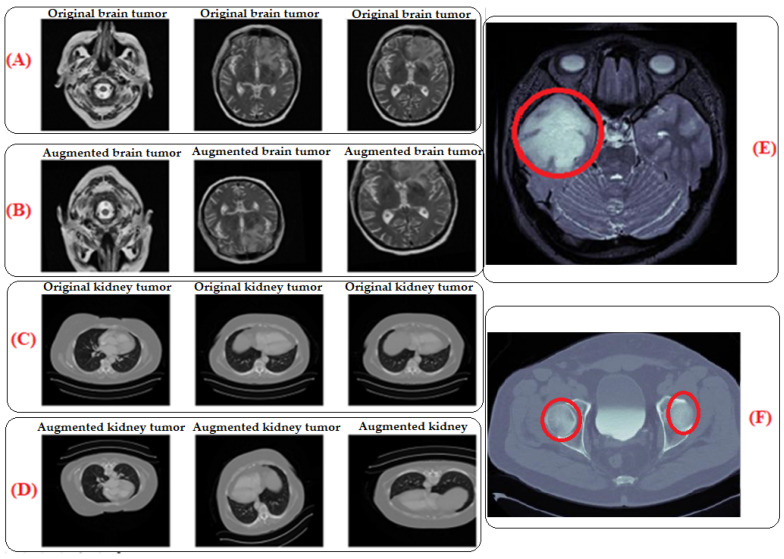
MRI images of original and augmented brain and kidney tumor data. The area circled in red color delineates the tumors. (A): Original brain tumor image (This represents the unaltered, initial image used in the training dataset, (B): Augmented brain tumor image (This corresponds to the image of the brain image that has undergone traditional data augmentation techniques), (C): Original kidney tumor image (This represents the original unaltered MRI scan focused on the kidney, (D): Augmented brain tumor image (This corresponds to the image of the kidney tumor, that has undergone traditional data augmentation techniques. (E): Magnified view of augmented brain tumor image, (F): Magnified view of augmented kidney tumor image.
